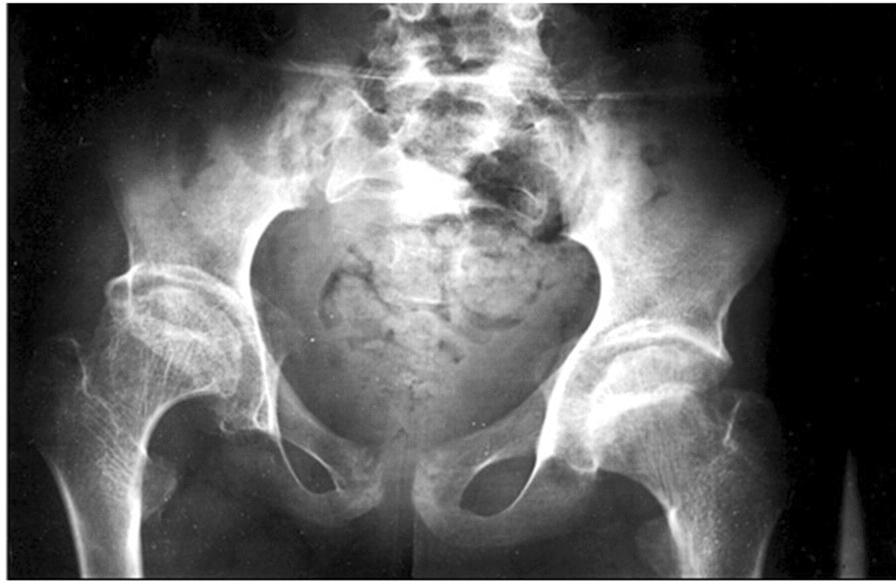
X-ray of the pelvis of the PPRD patient that reveals severe hip osteoarthritis
